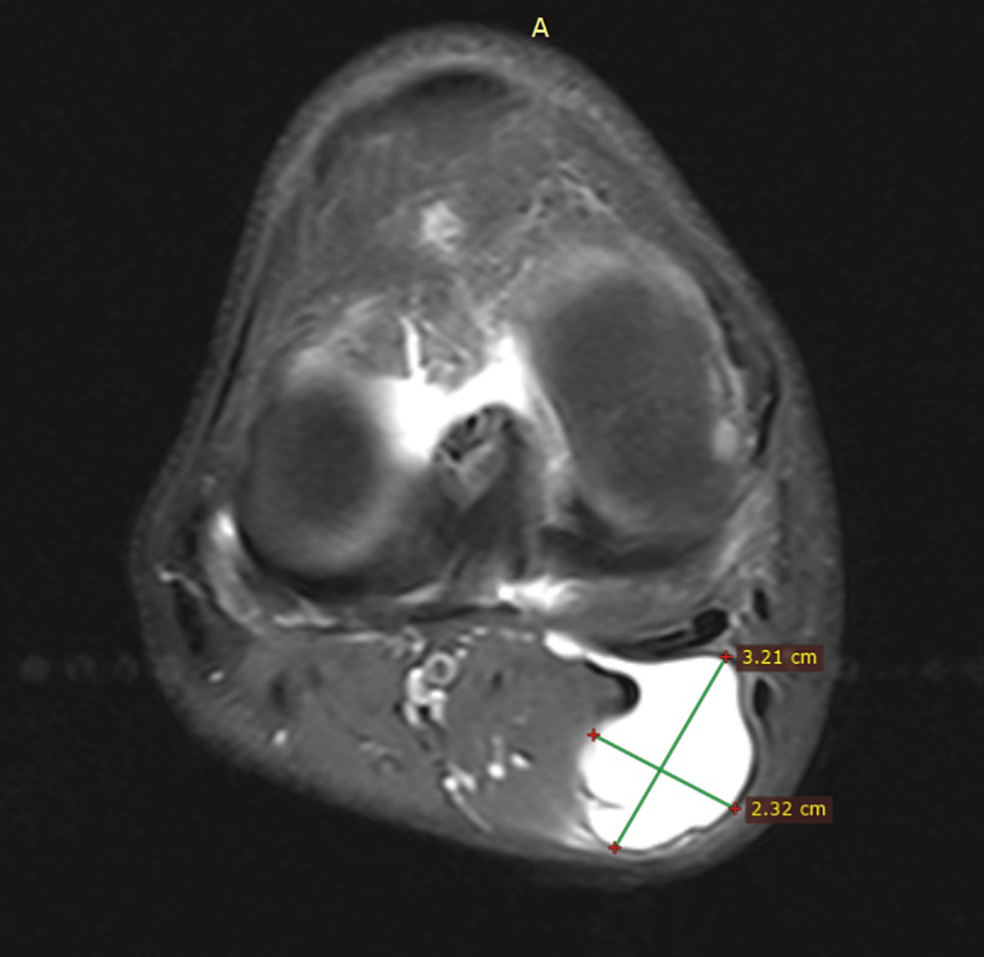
Axial T2-weighted image that shows the pedicle of the cyst adherent to posterior capsule. Selected sample MRI studies demonstrate the size of the cyst in relation to adjacent anatomical structures.
